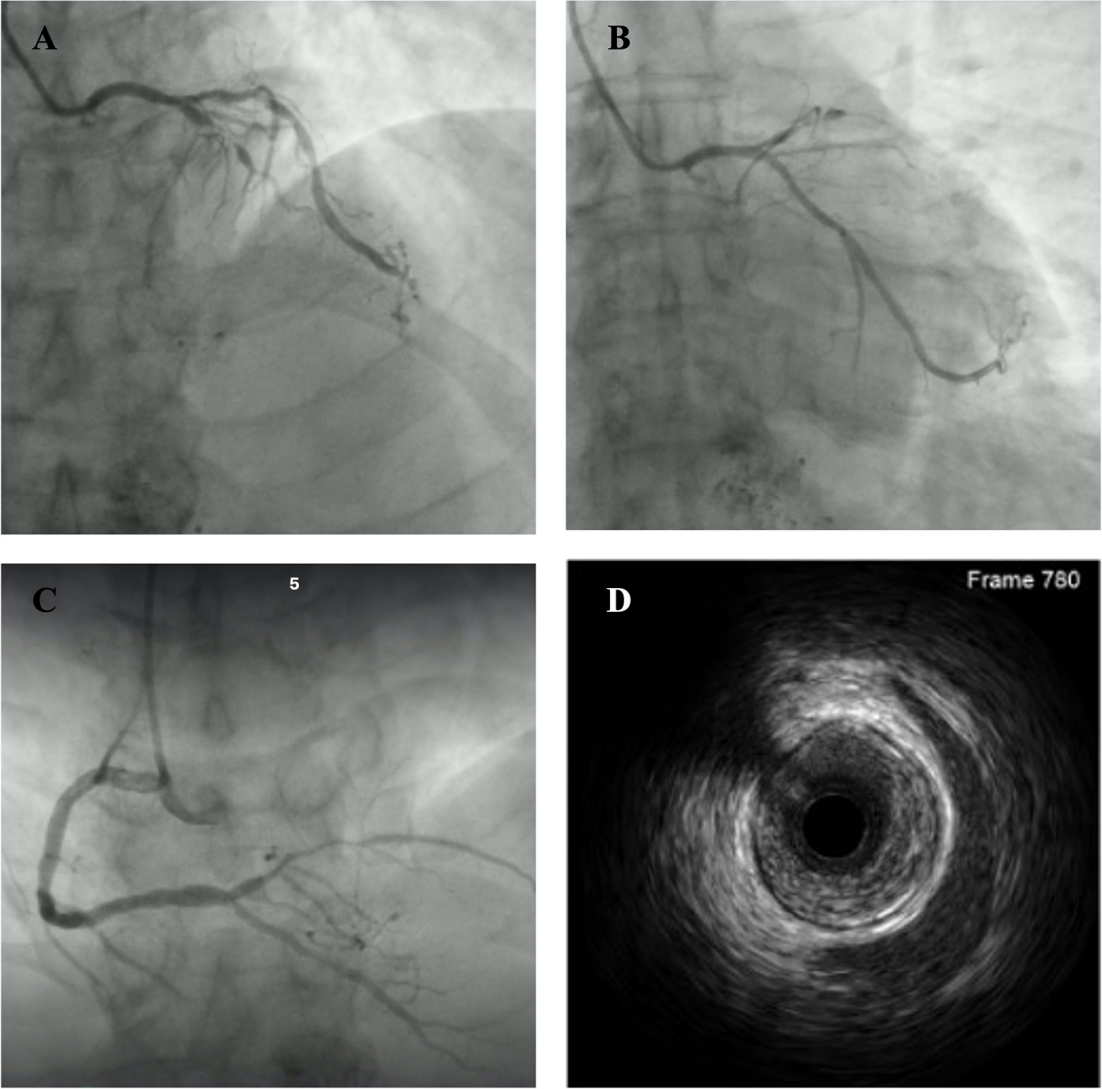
Baseline coronary angiography at first admission showed a subtotal occlusion of the left anterior descending artery with thrombolysis in myocardial infarction 0–1 flow (A), mild–moderate stenosis in the left circumflex artery (B), and right coronary artery (C). Intravascular ultrasound showed diffused atherosclerotic plaque in the left anterior descending artery (D).
